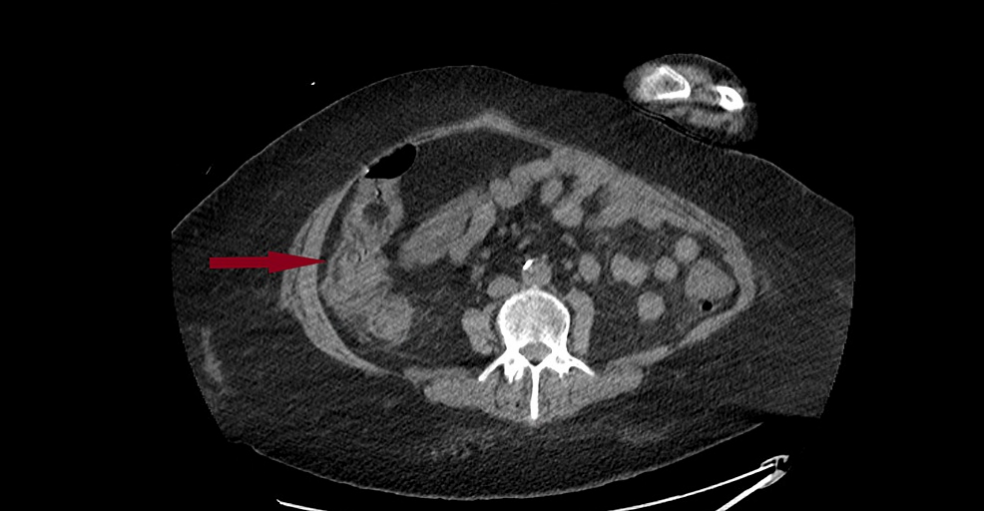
CT abdomen and pelvis without contrast: showing diffuse thickening of colonic wall with surrounding inflammatory changes. CT, computed tomography
